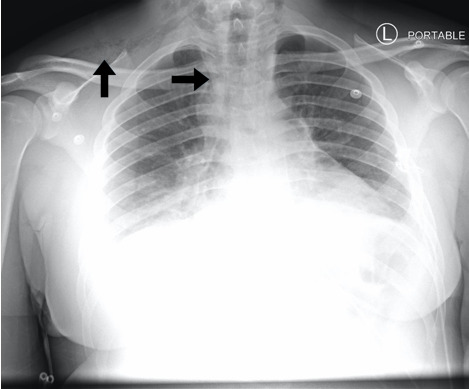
Chest radiograph demonstrating pneumomediastinum and subcutaneous emphysema
